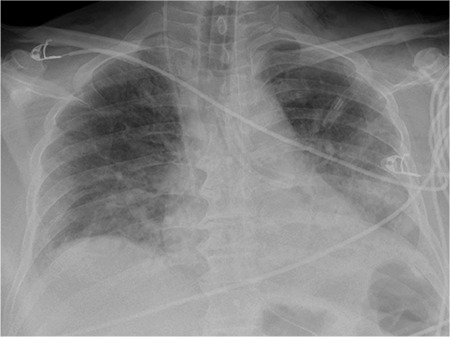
Chest radiograph showing the fractured tracheostomy tube in left main bronchus.
